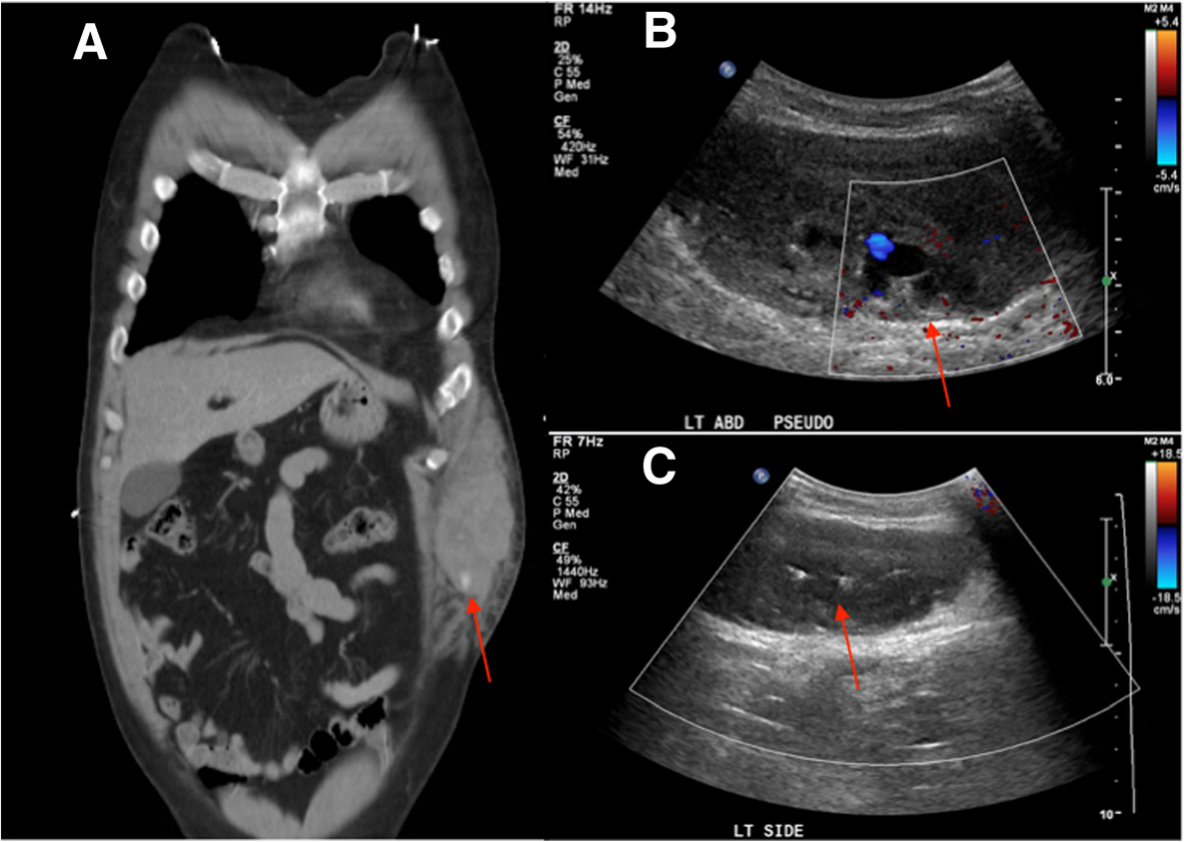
a Contrast enhanced CT of the abdomen and pelvis demonstrates a large left anterior abdominal wall hematoma with an area of active extravasation. b Ultrasound images with color Doppler through the left flank hematoma demonstrates focal pseudoaneurysm corresponding to the focus of active hemorrhage on the CT. c Short term follow up ultrasound image with color Doppler through the left flank hematoma demonstrates no residual pseudoaneurysm
